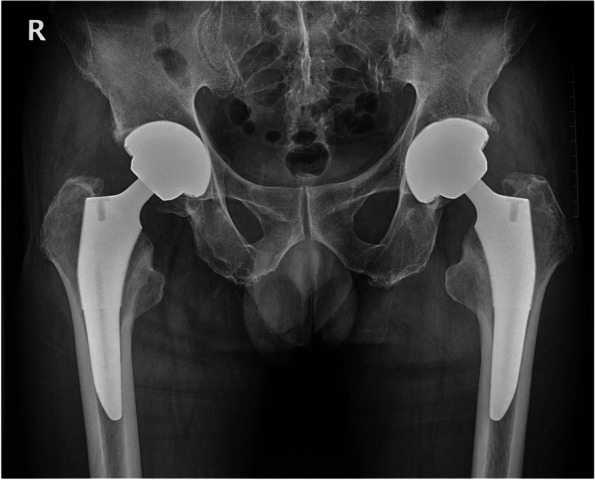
Plain radiograph of pelvis 15 months after right hip arthroplasty. Plain pelvic radiograph shows no protrusion of the ceramic liner. The liner remained properly seated after spontaneous reduction
